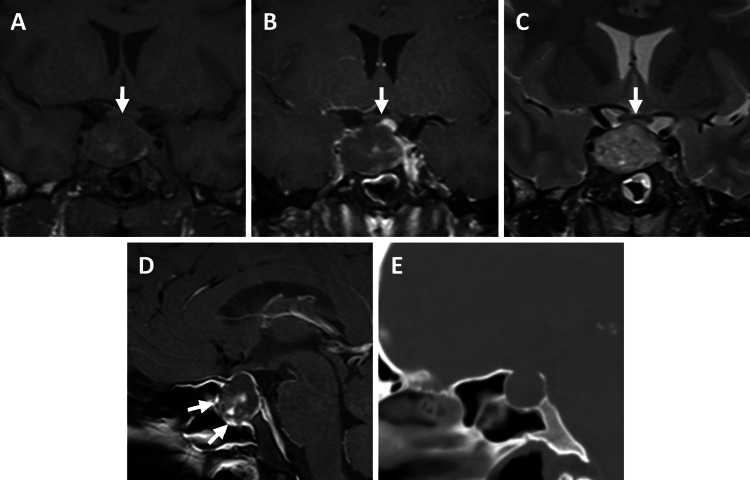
MRI and CT of the head demonstrated a 2.0×2.8×2.1-cm heterogeneously enhancing sellar and suprasellar mass Coronal T1-weighted noncontrast (A); T1-weighted postcontrast (B); and T2-weighted (C) MRI show upward displacement of the optic chiasm (white arrows) and internal blood products. The pituitary stalk and gland are displaced to the left, best seen on postcontrast T1-weighted imaging. Sagittal post-contrast T1 MRI (D) and CT (E) demonstrated expansion of the sella consistent with a prior slow-growing tumor. Enhancement of the sphenoid sinus mucosa is also seen (D; gray arrows), which has been linked to higher intrasellar pressures and more severe PA [8].
